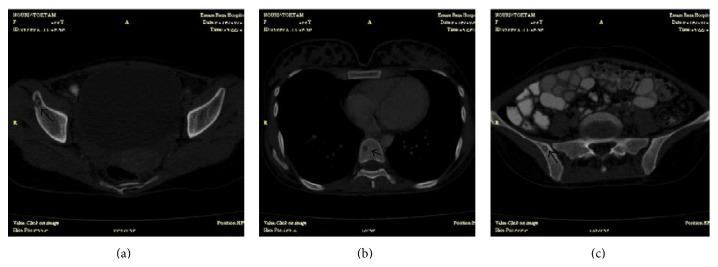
Computed tomography scan shows multiple small osteolytic lesions containing a sequestrum in acetabular roof (a), 8th thoracic vertebra (b), and ilium (c).
